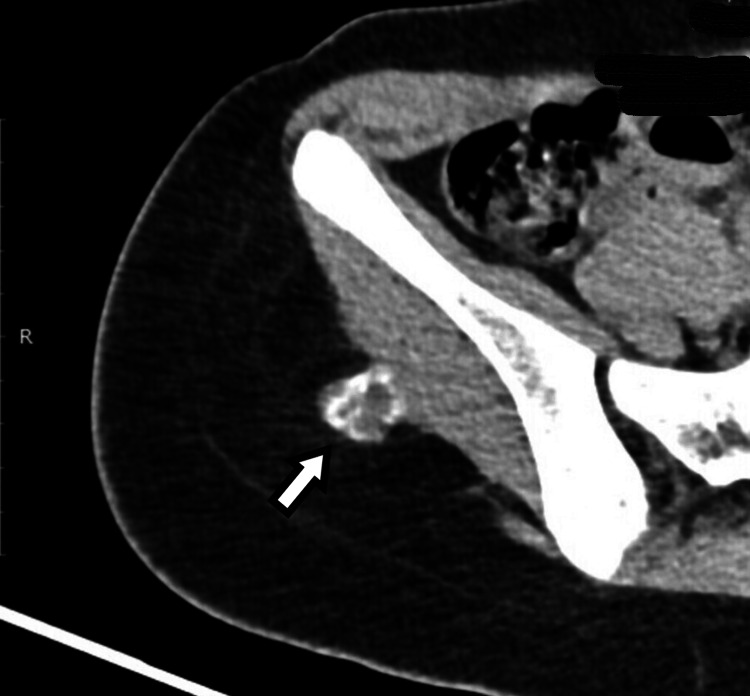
Axial CT scan after two months from the initial MRI shows a mass with peripheral ossifications
